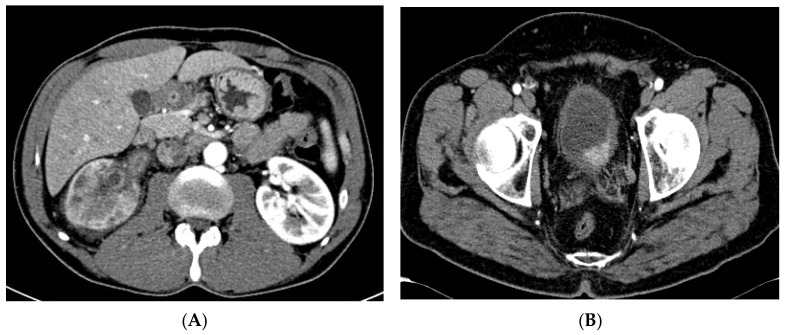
(A) Computed tomography (CT) image of a 75-year-old men with advanced right Upper Tract Urothelial Tumor (SMI value = 40.54 cm^2^/m^2^; (B) CT image of 72-year-old men with advanced Bladder Tumor on left posterior wall. (SMI value = 44.86 cm^2^/m^2^).
